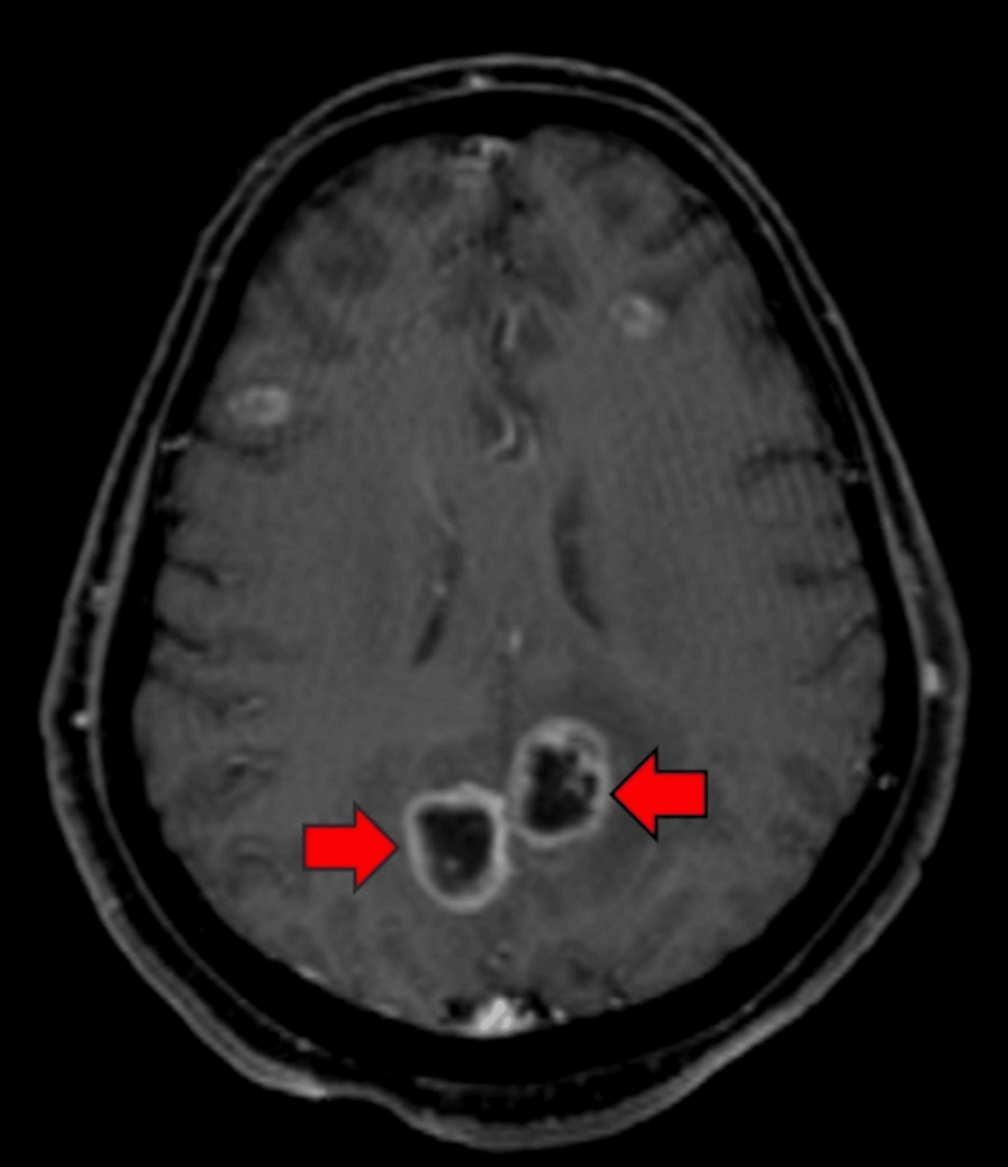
An axial MRI of the brain with contrast reveals multiple intra-axial, ring-enhancing T2 hyperintense lesions with surrounding vasogenic edema denoted by the red arrow.
